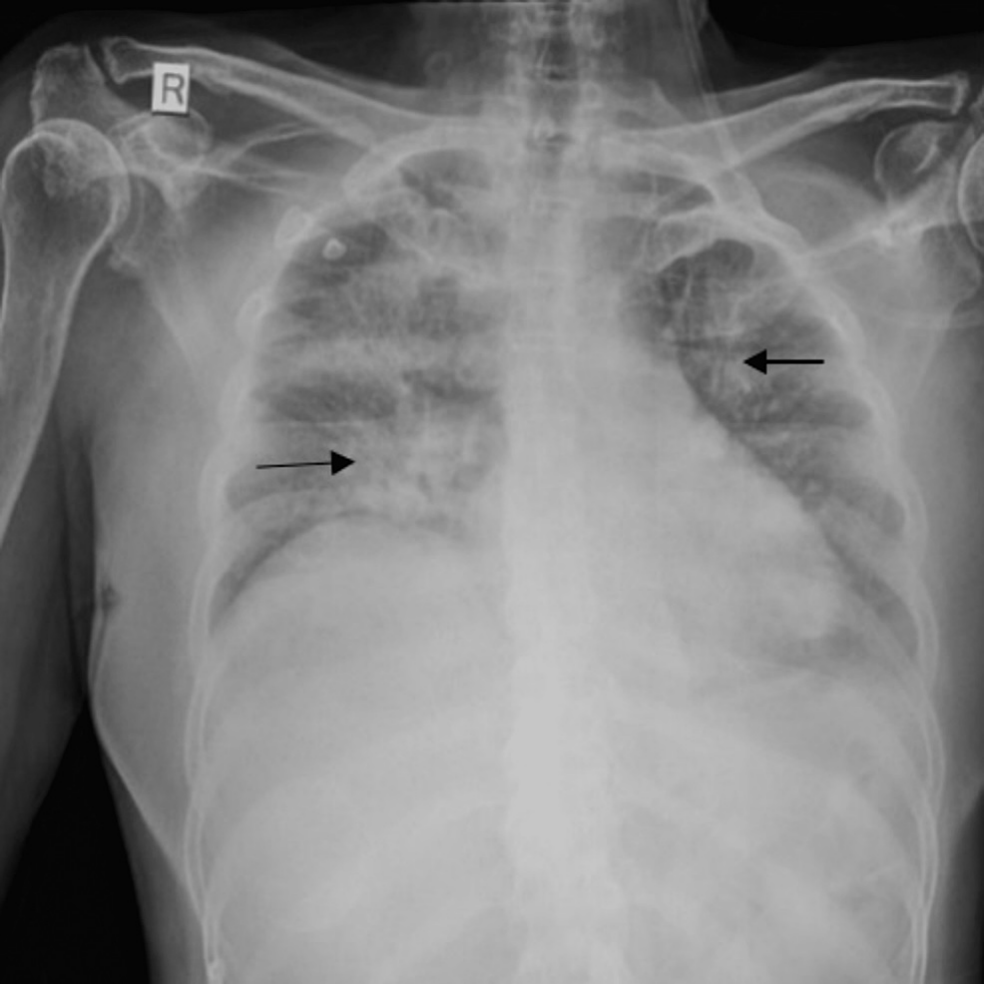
X-ray of the chest showing diffuse bronchopneumonia in right and left lungs (see arrows)
